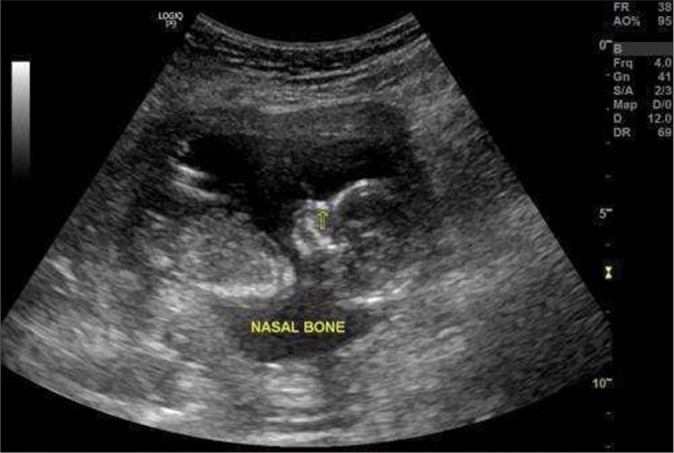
Sagittal gray scale ultrasound image of an intrauterine fetus of gestational age 16 weeks, 3 days showing the measurement method of fetal nasal bone (arrow) as described by Sonek *et al.*^[5]^
